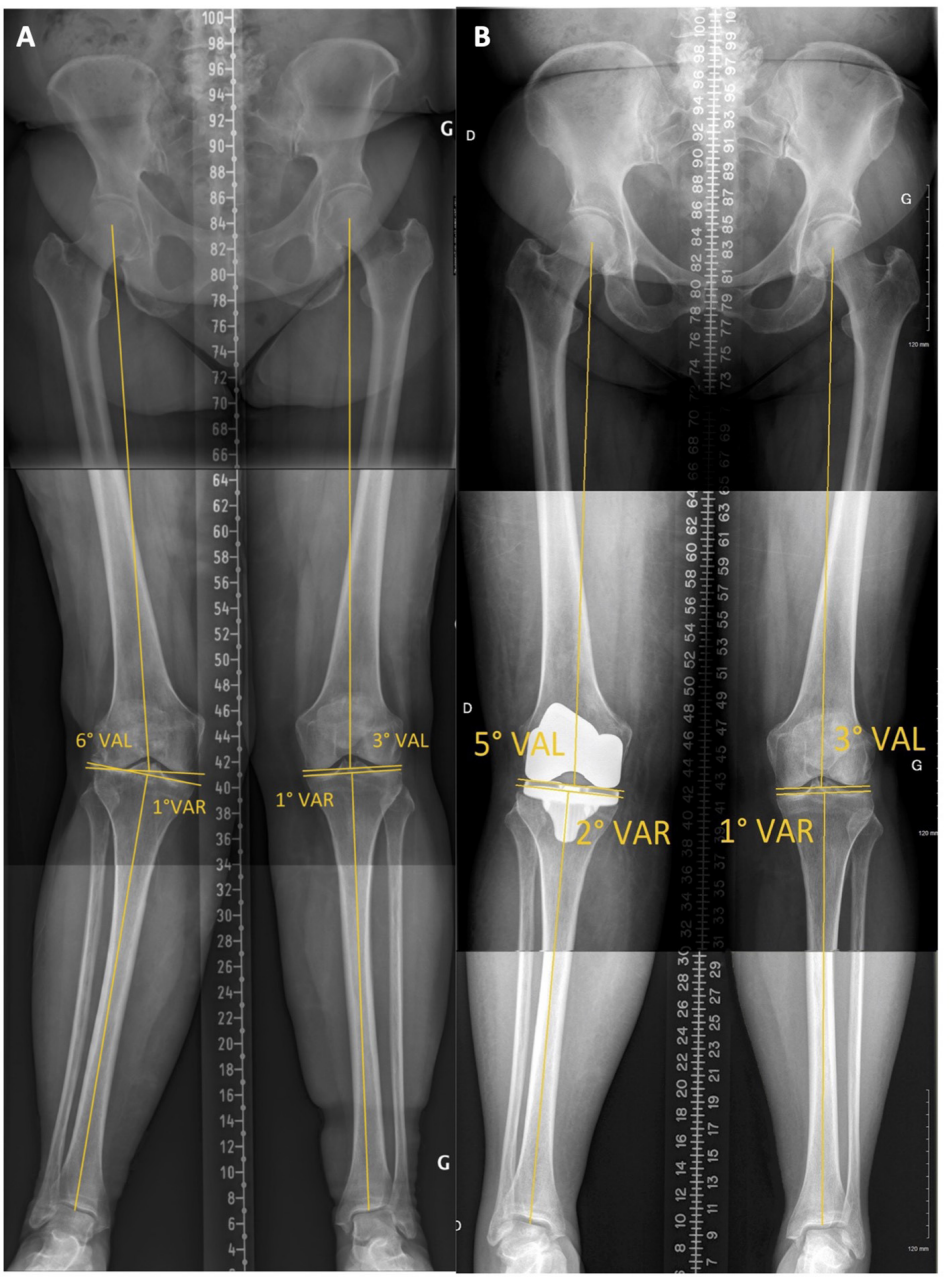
Case example 2, (A) preoperative standing long radiograph of a patient with a left lower limb mDFA of 6° valgus and a mPTA of 1° varus, resulting in an aHKA of 5° valgus. (B) on the postoperative radiograph, following the rKA algorithm, the implants mDFA is 5° valgus and mPTA is 2° varus.
